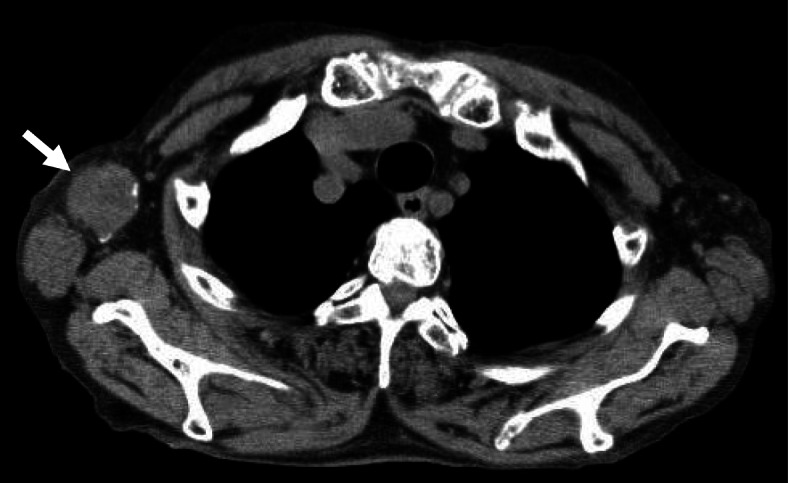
Findings of CT performed in October 2019. A solitary swollen lymph node measuring 37 × 33 mm in size with small high-density spots in the peripheral region was observed in the right axillary region (arrow)
